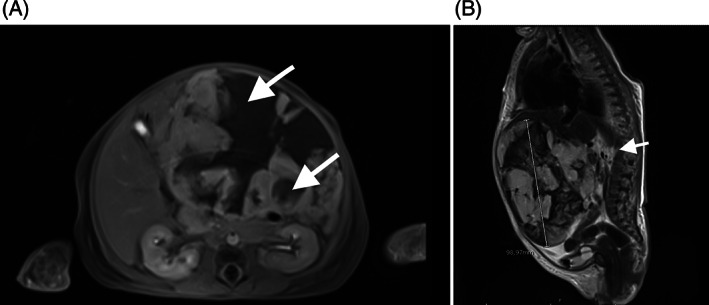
(A, B) Abdominal magnetic resonance image of hepatic hemangioendothelioma in case 3. The mass had with necrotic areas in the center (arrows). The hepatic origin of the mass is unclear in the images, which instead shows it originating from the retroperitoneum
